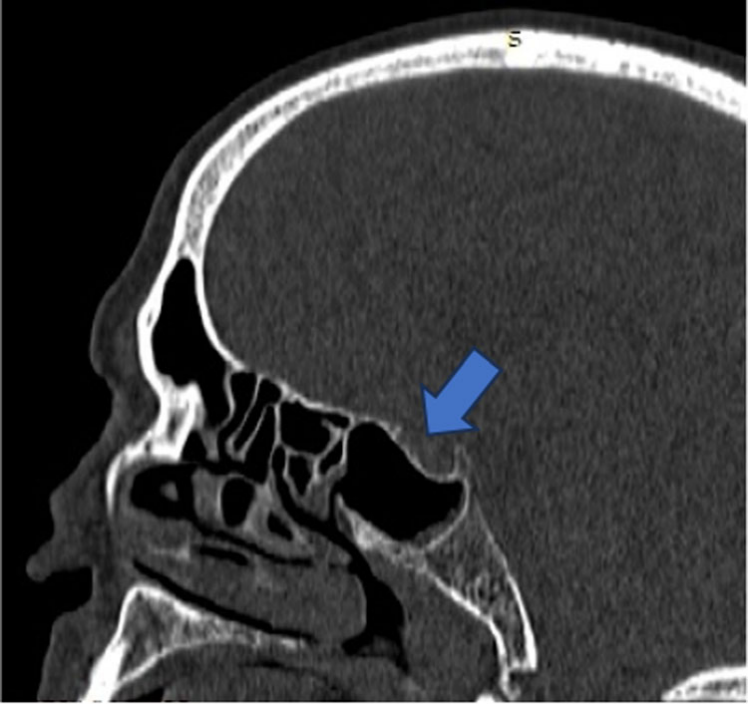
CT Sagittal image shows post‐sellar pneumatization of sphenoid sinus.
